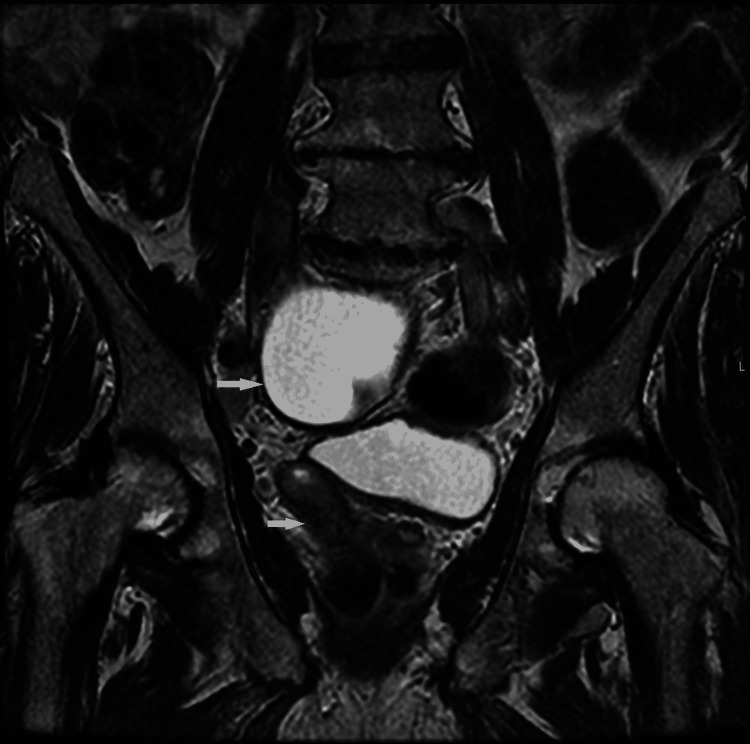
MRI Pelvis (Coronal View) confirming Zinner Syndrome Note that there is no local lymphadenopathy or bony metastasis
